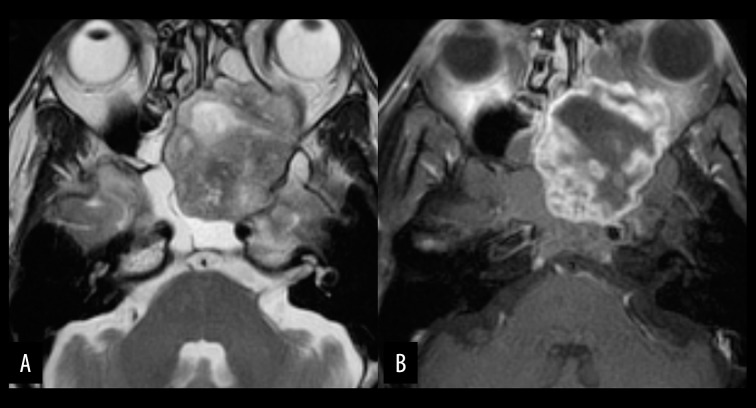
Chondrosarcoma of the sphenoid in a 56-year-old man. (A) Axial, T2-weighted MR image shows a lobulated mass at the sphenoid (arrows). The mass contains abundant intermediate signals but also includes hyperintense foci. (B) Axial, contrast-enhanced, MR image with fat suppression shows peripheral enhancement that indicates chondrogenic tumor. A pathological analysis revealed grade 1 chondrosarcoma.
